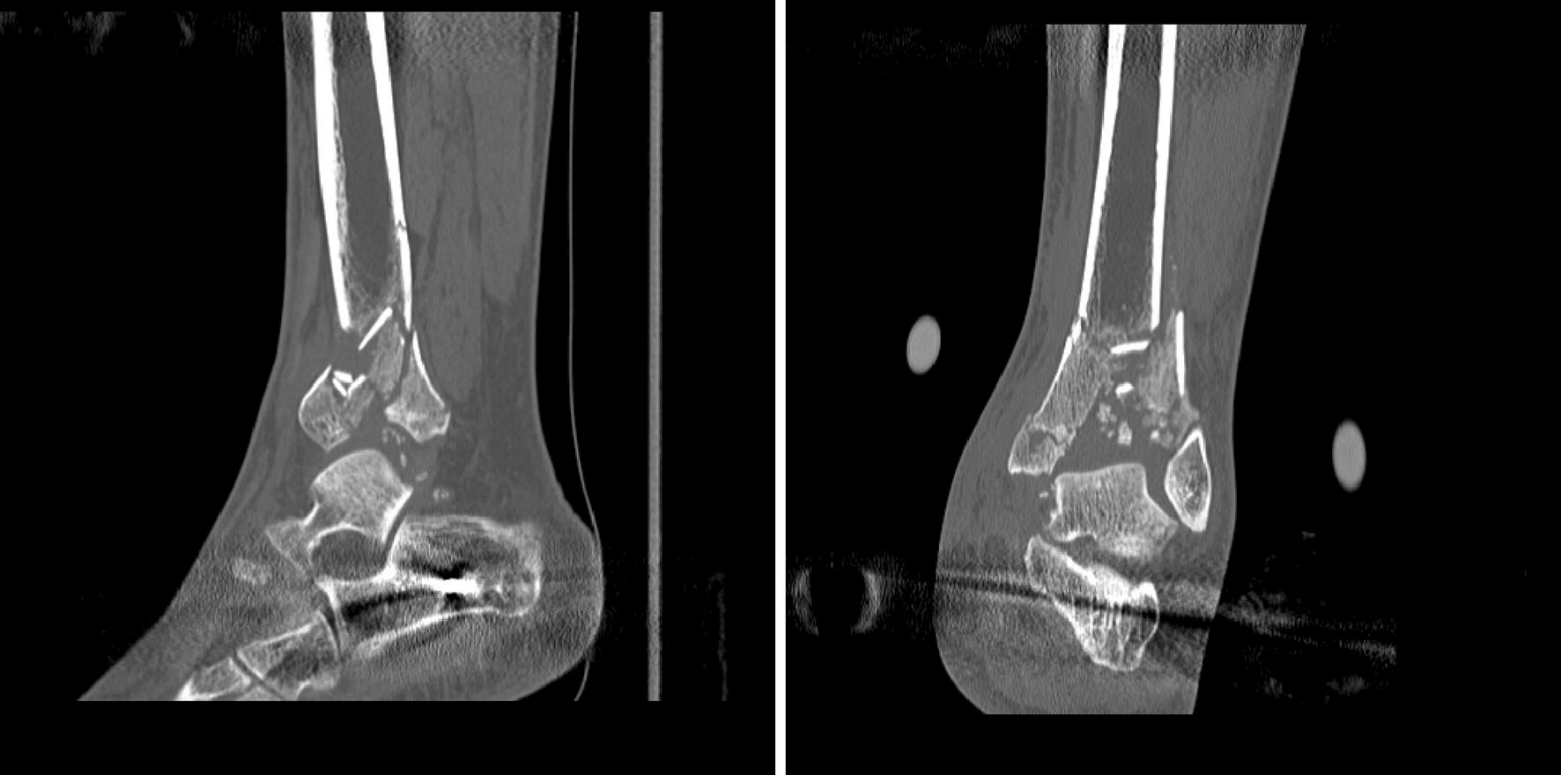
Anterior and central areas are most often comminuted, and central die-punched fragments can be appreciated on both axial and reformatted sequences on CT
